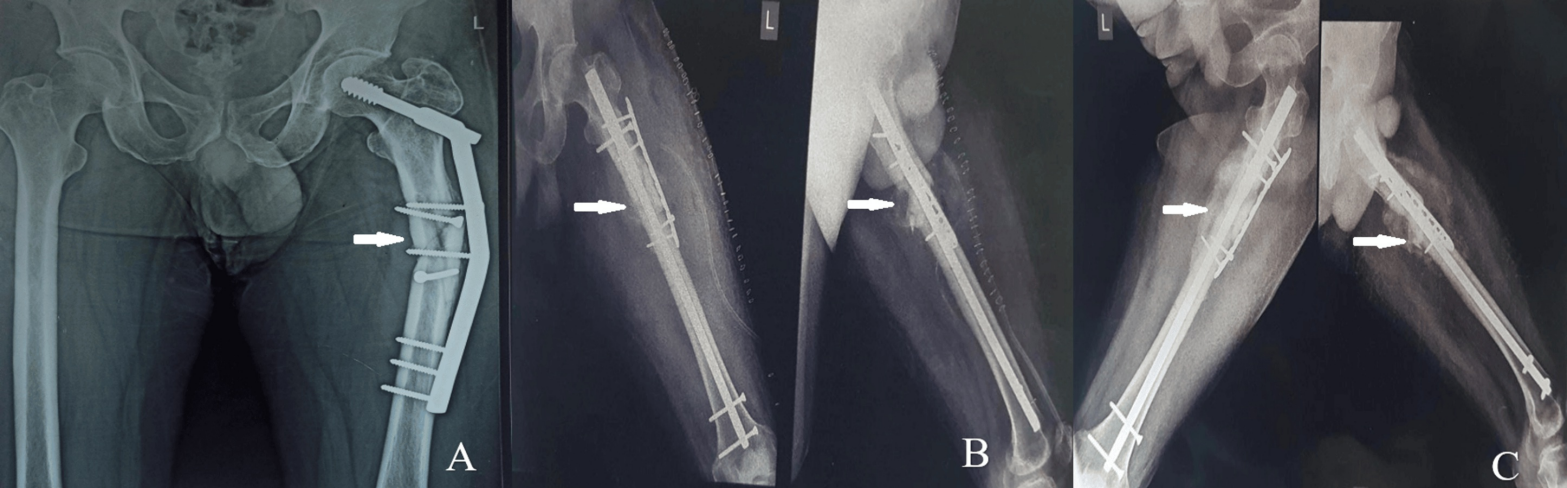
A 54-year-old male with implant failure and nonunion of left femur proximal 1/3rd shaft fracture repaired with a dynamic hip screw. A. Preoperative radiograph taken nine months after dynamic hip screw fixation, showing implant failure and nonunion. B. Immediate postoperative radiograph following IMN with DCP plate and graft augmentation. C. A radiograph taken three months postsurgery demonstrates bone union with satisfactory consolidation observed at the fracture site. IMN, intramedullary nailing; DCP, dynamic compression plate
